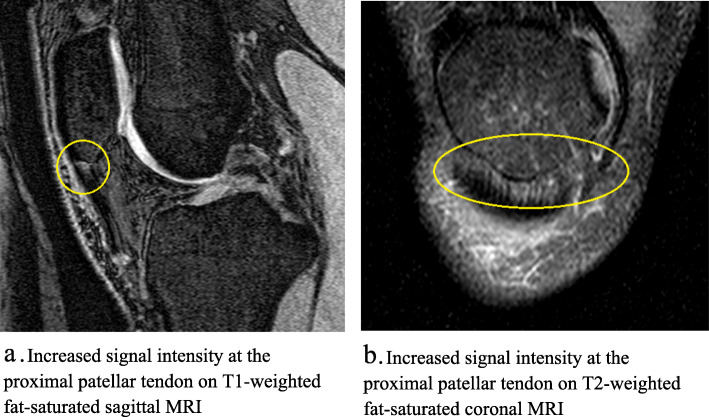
Increased signal intensity at the proximal patellar tendon on a. T1-weighted fat-saturated sagittal MRI; and b. T2-weighted fat-saturated coronal MRI
